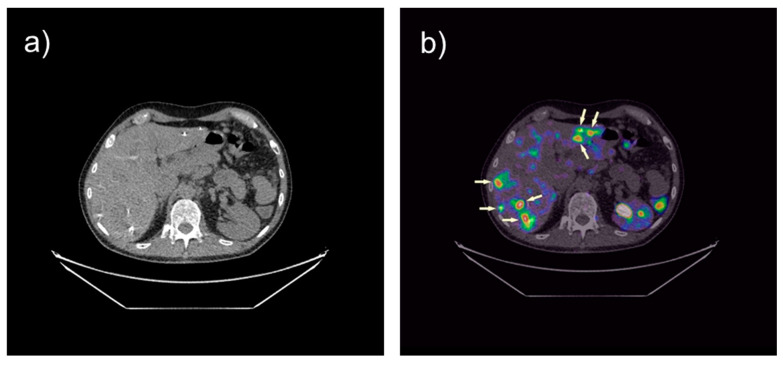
CT (a) and fused [^68^Ga]Ga-DOTA-MGS5 PET/CT (b) images in transverse orientation. Multiple liver metastasis (arrows) in a male patient with advanced MTC are visible; images performed 4 h after injection of 165 MBq (<50 µg peptide); courtesy of Prof. Alicja Hubalewska-Dydejczyk.
